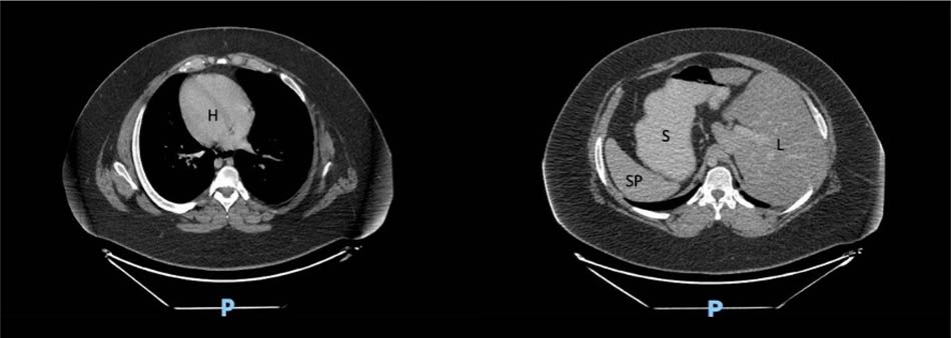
Computed tomography scan of the abdomen in our patient with SIT showing dextrocardia (H), spleen (SP) and stomach (S) located on the right side and liver (L) on the left side.
